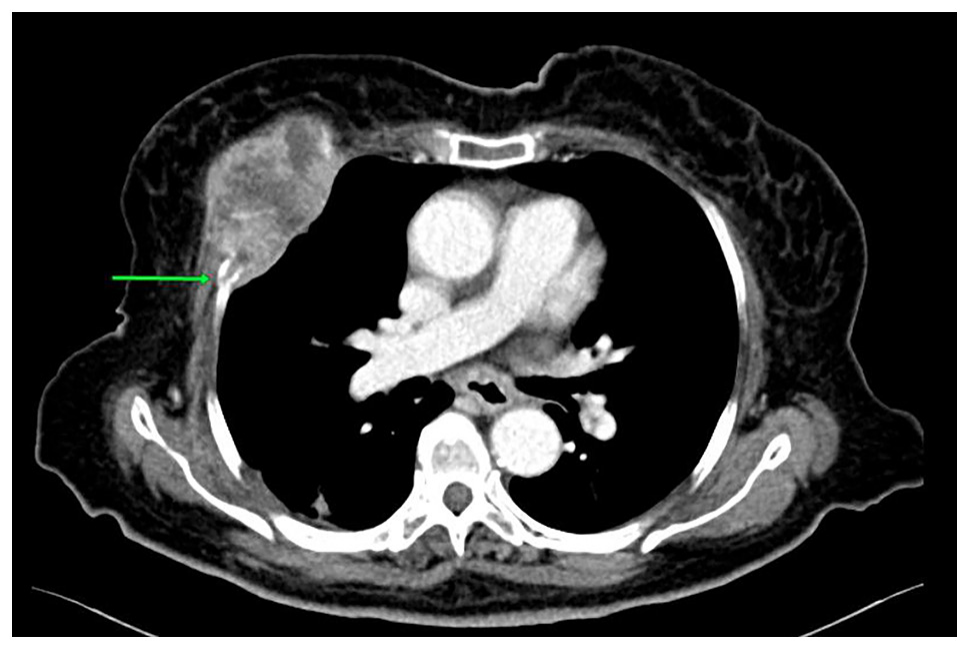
A contrast enhanced computed tomography scan of the chest, axial section. A heterogeneous enhanced soft tissue destructive mass on the anterior part of the right fourth rib (green arrow) was observed, consistent with bone metastasis.
